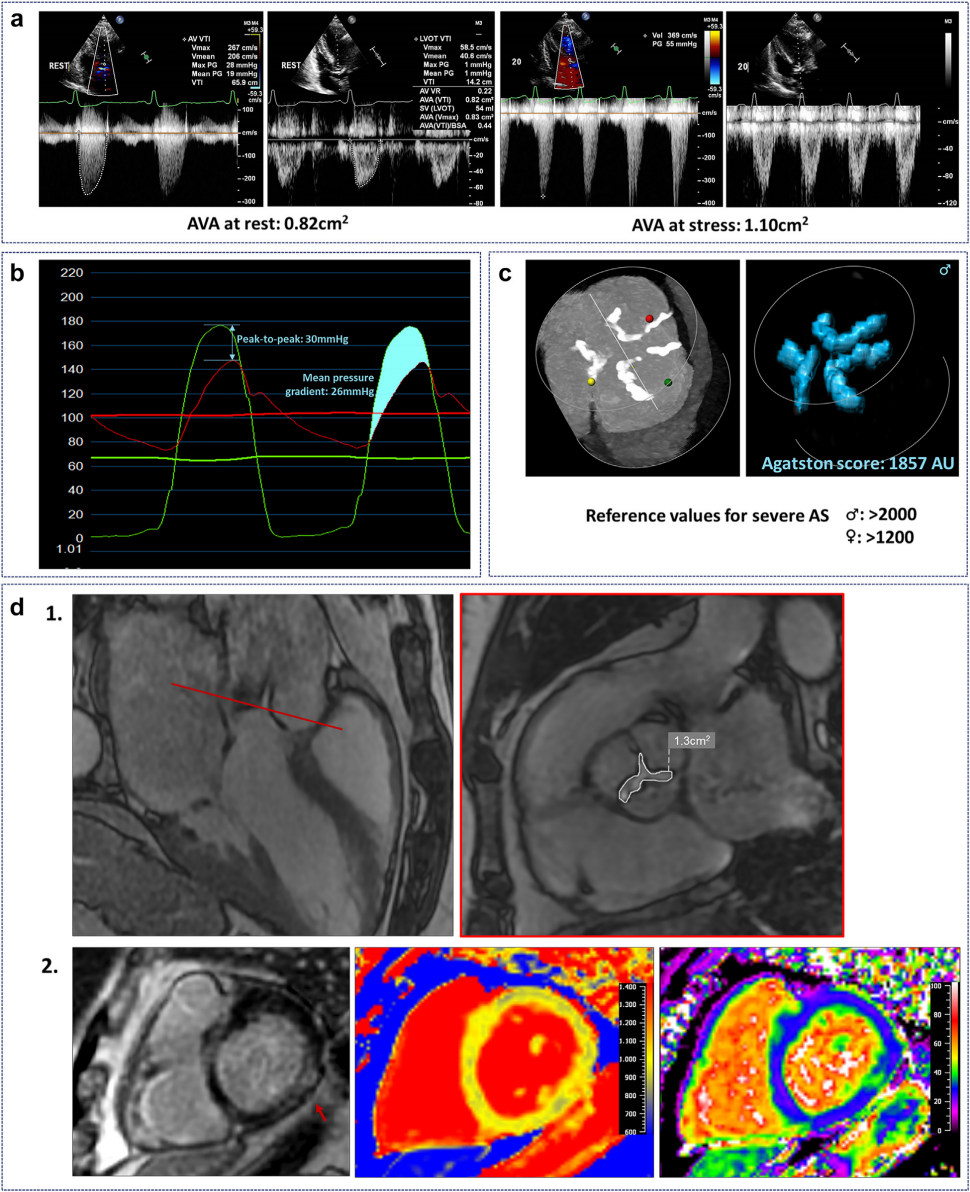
Imaging modalities in the diagnosis and evaluation of moderate aortic stenosis and associated left ventricular changes. (a) dobutamine stress echocardiography. Low peak aortic valve velocity and mean pressure gradient at rest in combination with aortic valve area <1.0 cm^2^ indicate low-flow low-gradient aortic stenosis. Dobutamine stress increases aortic valve area >1.0 cm^2^, indicating pseudo-severe, or moderate, aortic stenosis. (b) Aortic valve calcification in a male patient showing Agatston score of 1857 arbitrary units, corresponding to moderate aortic stenosis. (c) invasive pressure measurements showing measurements fitting with moderate aortic stenosis. (d.1) left: systolic cine image in 3-chamber view; and right: short axis aortic valve with aortic valve area measurement at level of red line in left. (d.2) left: late gadolinium enhancement image of the basal short axis with mid-wal enhancement inferolateral (red arrow). Middle: short axis T1 map. Right: extracellular volume map at the level of the papillary muscles with normal native T1 and extracellular volume in the septum and increased T1 and extracellular volume in the area with late gadolinium enhancement. Abbreviations: AS, aortic stenosis; AU, Agatston units; AVA, aortic valve area.
